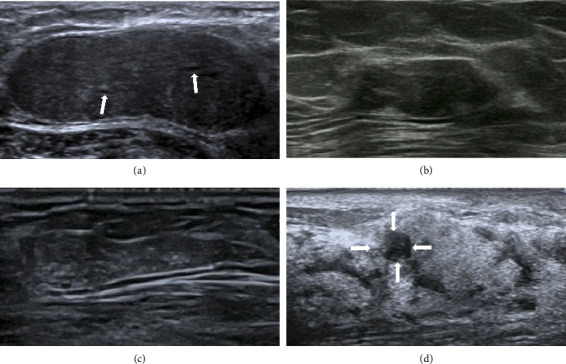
(a) Conventional ultrasound suggests BI-RADS 3, with clear margins, regular morphology, and linear hypoechoic structures within the lesion (”↑“); (b) conventional ultrasound suggests BI-RADS 4a, with indistinct margins and somewhat irregular morphology; (c) conventional ultrasound indicates BI-RADS 4b, with indistinct margins, irregular morphology, and internal punctate hyperechoic foci; and (d) conventional ultrasound indicates BI-RADS 4c, with indistinct margins, irregular morphology, and internal punctate hyperechoic foci, and the lesion demonstrates nonmass-like changes. It solely suggests a hypoechoic lesion (“↑”) mixed with the breast parenchyma.
